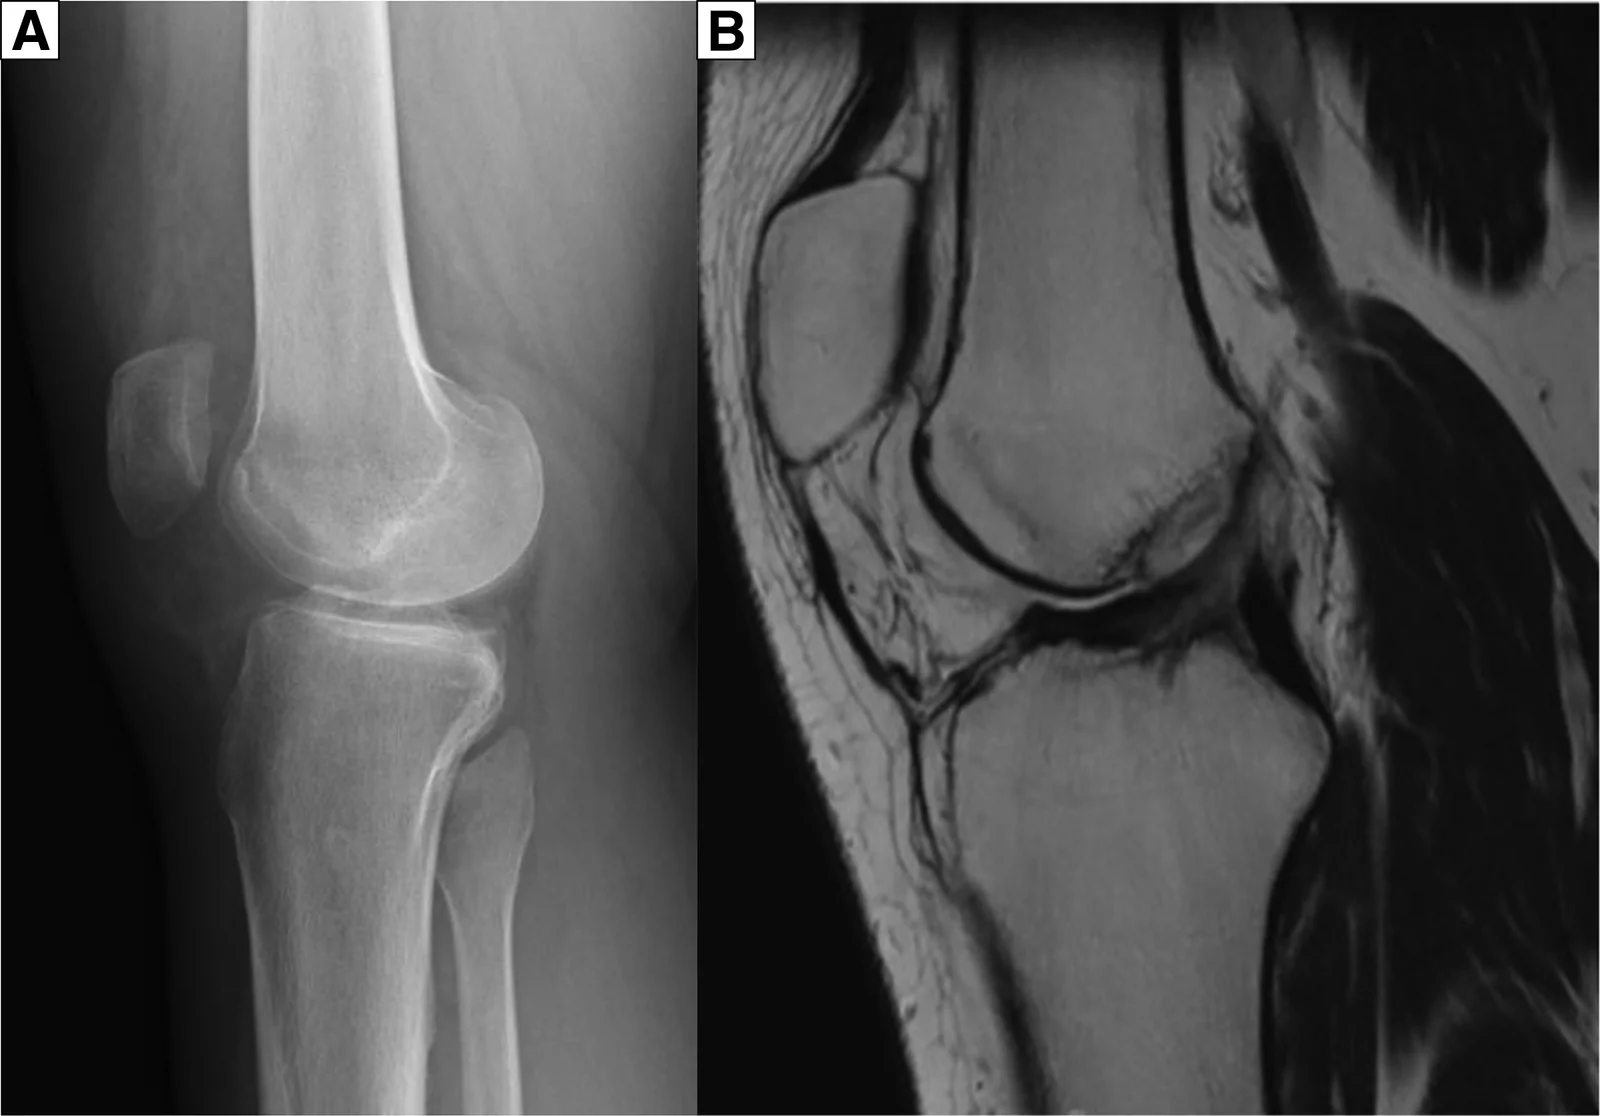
Preoperative imaging of the left knee. (A) Lateral radiograph demonstrating patella alta with an Insall–Salvati ratio of 1.28. (B) Sagittal magnetic resonance image confirming complete disruption of the patellar tendon.
